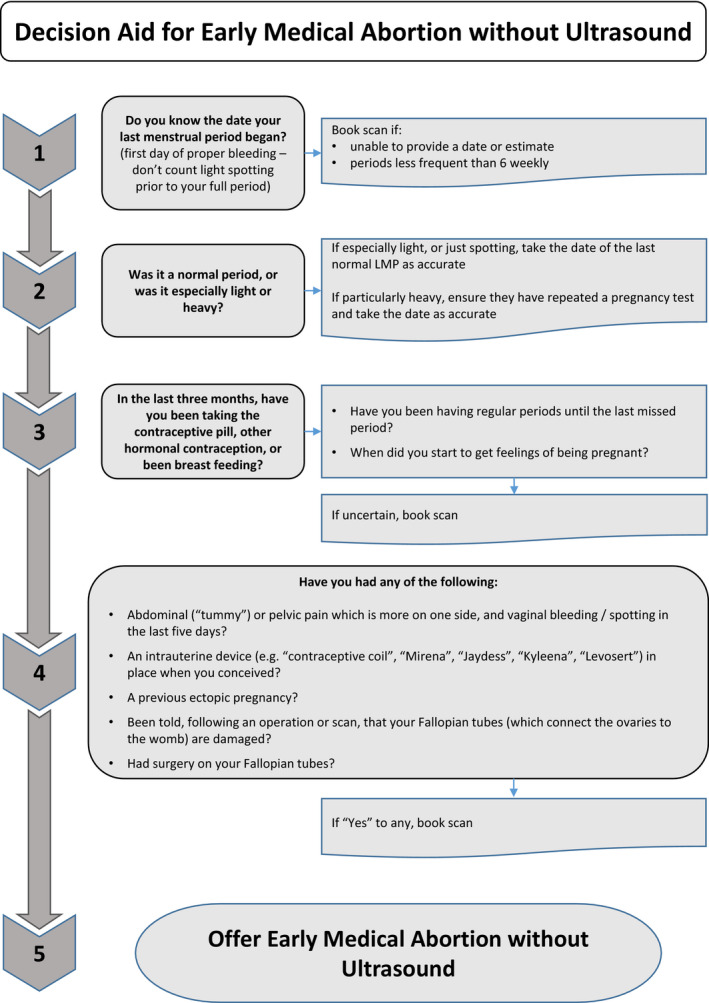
Decision aid for early medical abortion without ultrasound (adapted with permission from RCOG Coronavirus (COVID‐19) infection and abortion care – information for healthcare professionals; 2020‐06‐04‐decision‐aid‐for‐early‐medical‐abortion‐without‐ultrasound.pdf [rcog.org.uk]^17^)
